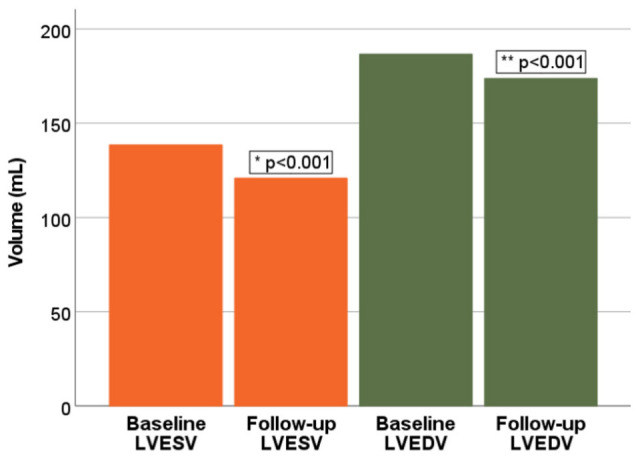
Echocardiographic data. LVESV, left ventricular end systolic volume. LVEDV, left ventricular end diastolic volume.
